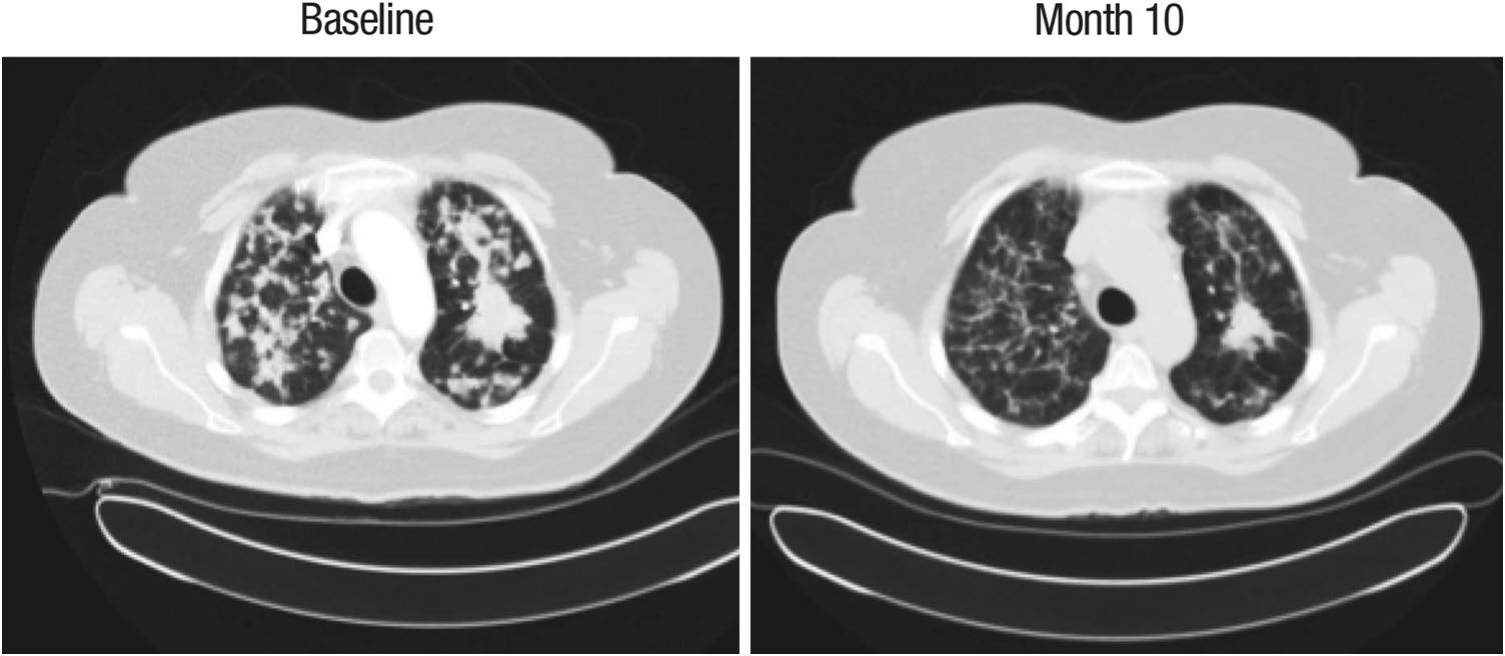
Computed tomography images showing an unconfirmed partial response in a patient with NSCLC who received afatinib 30 mg, paclitaxel 80 mg/m^2^, and bevacizumab 5 mg/kg. This patient received afatinib for 629 days. A partial response was reported twice in this patient during the study; however, these are considered to be unconfirmed partial responses because, at the consecutive tumor assessments, the percentage change in tumor lesions did not meet RECIST v1.0 criteria for partial response
